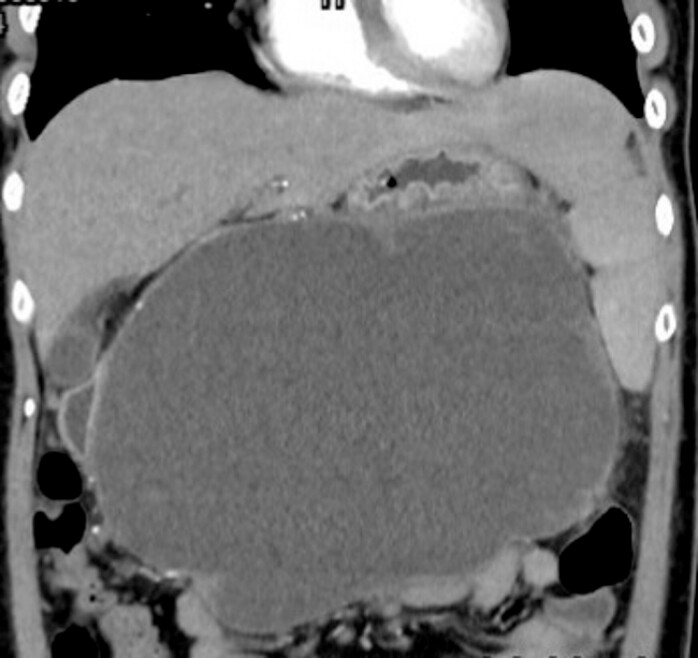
Computed tomography revealed a large pancreatic pseudocyst.
